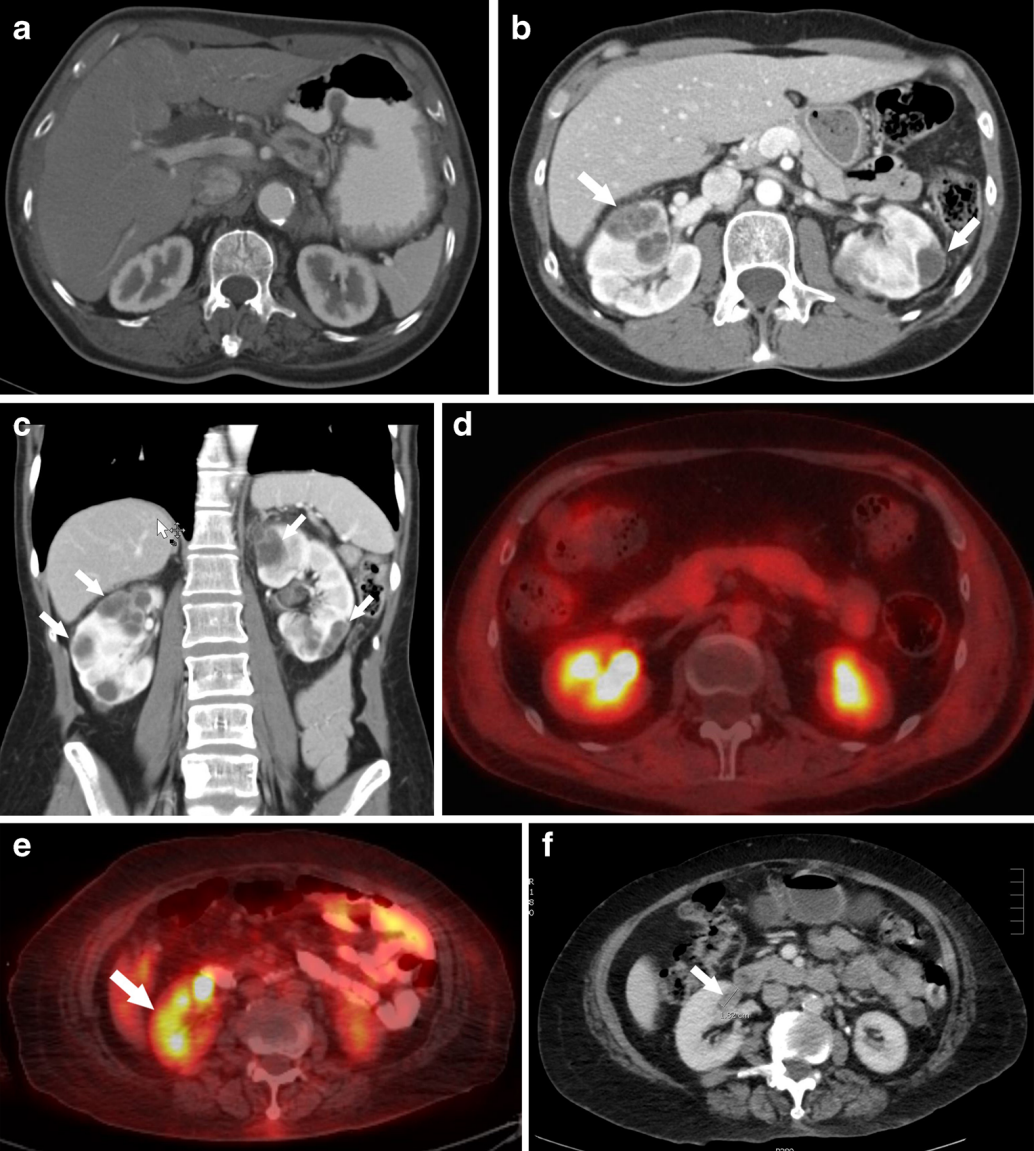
Forty-nine-year-old female with NSCLC being treated with long-term crizotinib therapy. (a) Pre-treatment axial CT with normal renal findings. (b, c) Axial and coronal CT images, respectively, performed 3 years following initiation of treatment with crizotinib revealing multiple complex cystic lesions bilaterally (*arrows*). (d) Axial positron emission tomography (PET)/CT without evidence of abnormal FDG-avid uptake supporting a benign process. (e, f) Axial PET/CT of a different patient with abnormal FDG-avid uptake positive for renal neoplasm provided as comparison (*arrows*)
